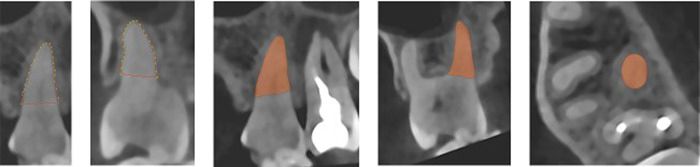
Segmentation and control of the accuracy of the suggested root shape in the three orthogonal planes (sagittal, coronal, and axial) in comparison to the root shape in the CBCT image. CBCT, cone‐beam computed tomography
